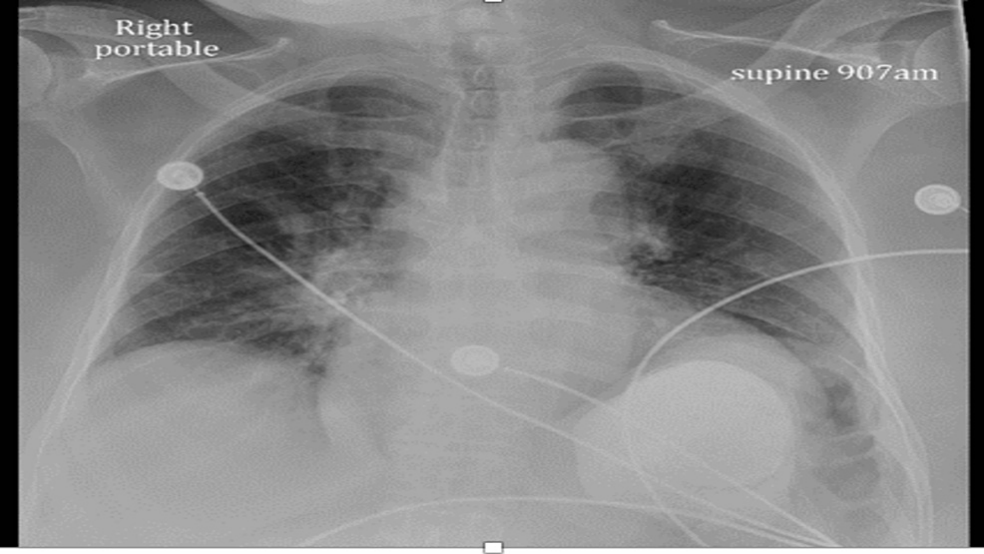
Chest X-ray showing a patchy left upper lung pulmonary opacity and patchy bibasilar subsegmental atelectasis
